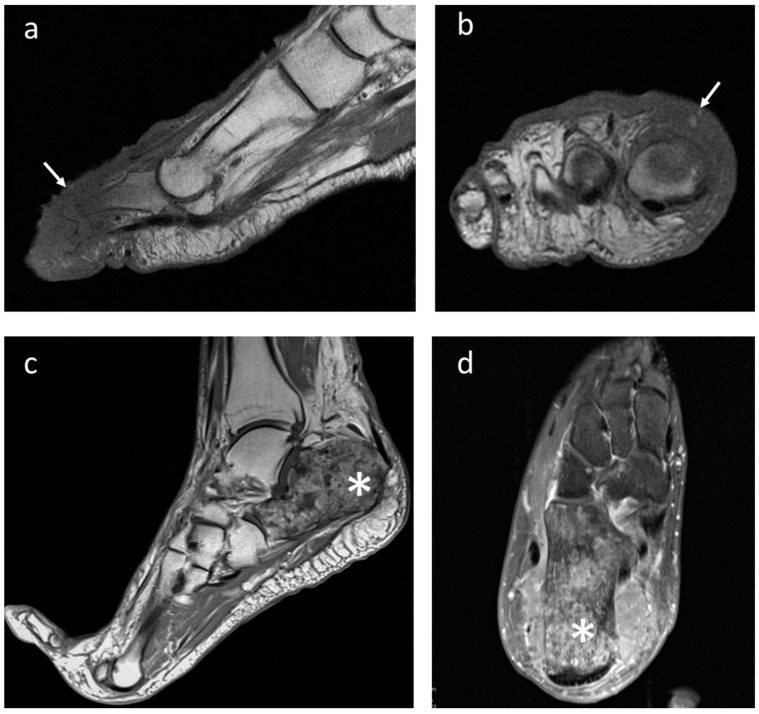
Right forefoot metastasis in a female patient with anaplastic large cell lymphoma (white arrows). Imaging appearance at MRI in (a) sagittal and (b) coronal projection; (c) metastasis from melanoma showing a full necrotic appearance of the calcaneus (asterisk) on T1-weighted sagittal and (d) transverse T2-weighted sequences.
